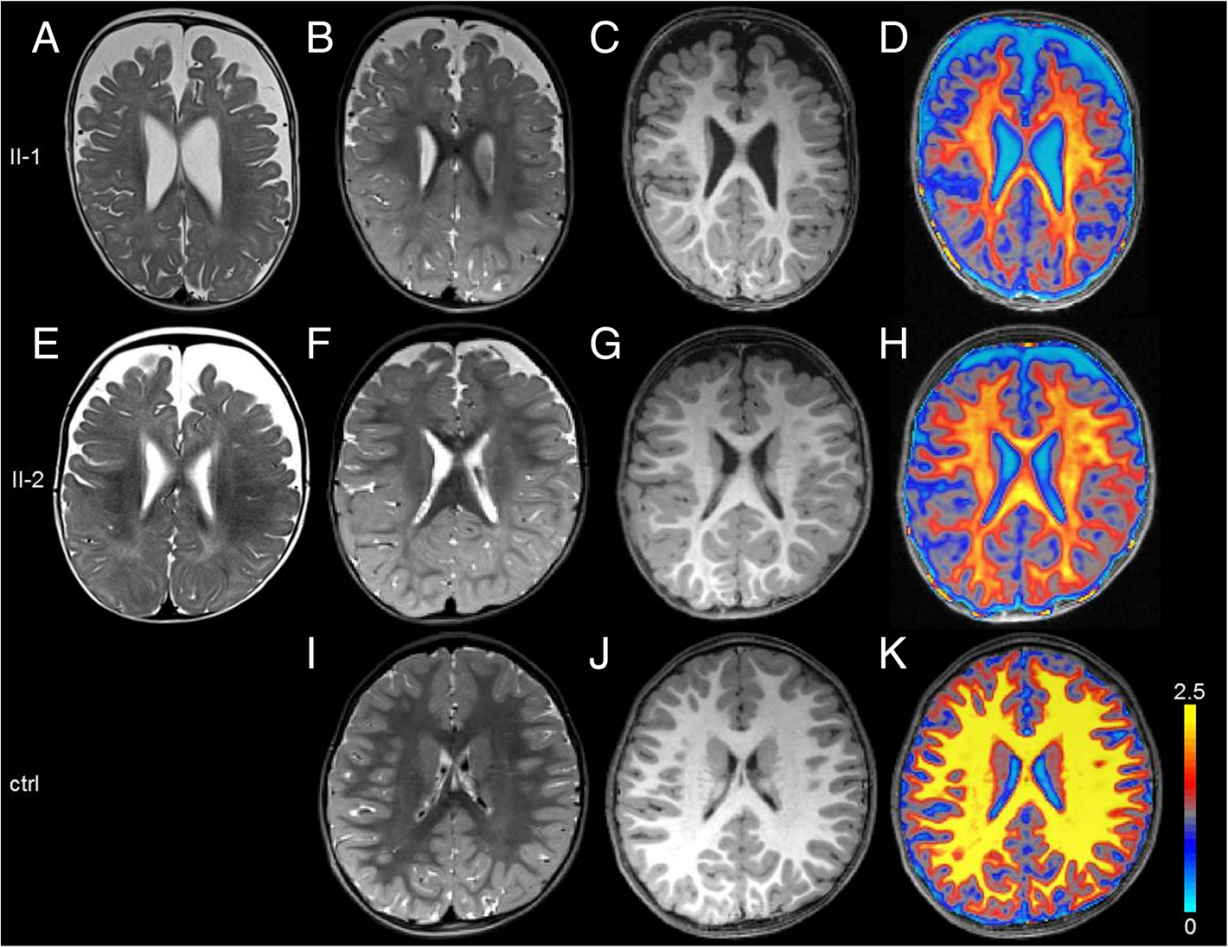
Structural and quantitative MRI of patients and a healthy age matched control. a;b;e;f;i: T2-weighted images at the corrected age of 12 months (a, e) and 2 years (b, f, i) of patients II-1 (a, b) and patient II-2 (e, f) and control (i) showing brain atrophy and significant delayed myelination in the patients. c;d;g;h;j;k: T1-weighted images and overlay of color coded MT saturation maps onto the corresponding T1-weighted images of both patients (c, d, g, h) and an age matched control (j, k) at age 2 years. Yellow represents high signal intensity considered to arise from properly myelinated WM. Both patients show widespread marked reductions of the myelinsensitive parameter MT saturation [12] when compared to the age matched control thereby emphasizing the delayed myelination. For detailed information about MT saturation maps see Additional file 1
